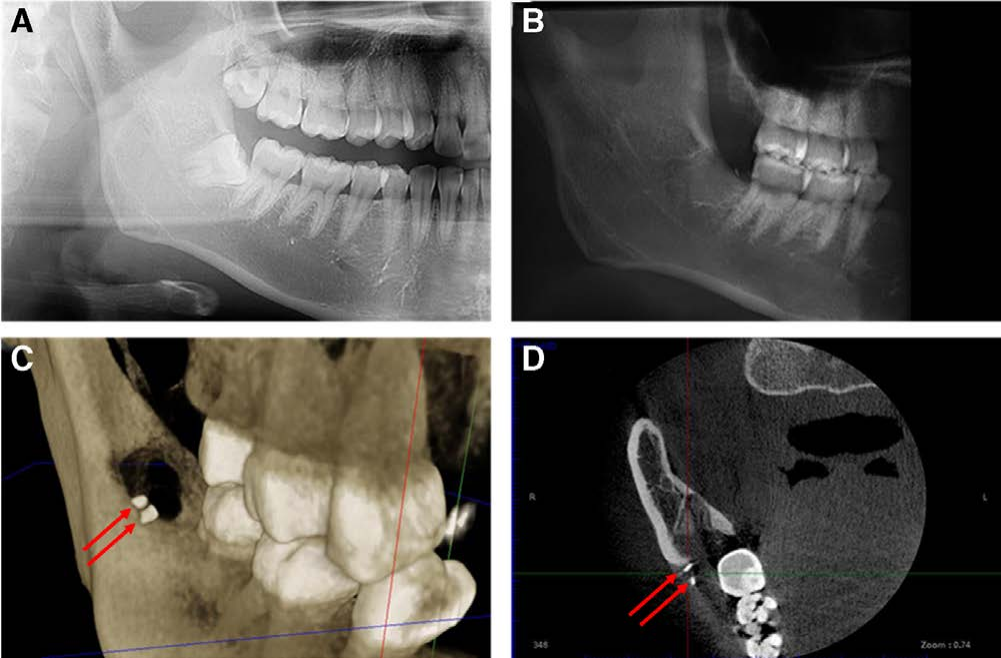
(A) The orthopantomogram of the patient’s impacted tooth on the right mandible before pre-extract; (B) The cone-beam computed tomography after tooth extraction; (C) The reconstructed image after tooth extraction; (D)The horizontal plane of the retained tooth structures.
